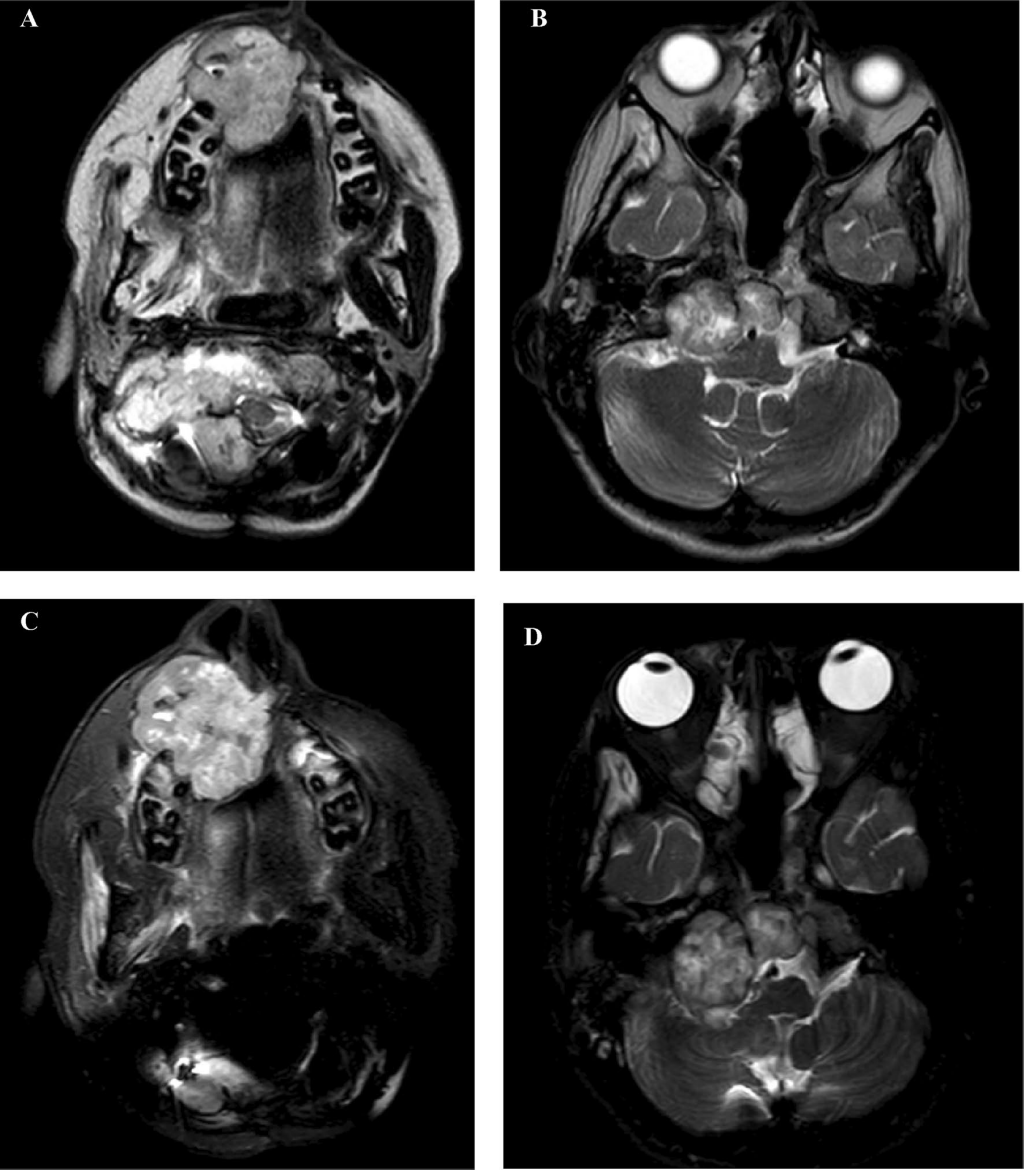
Case 3 MRI Brain. Axial T2 MRI sequences of patient with chordoma; (A,B) before starting pemetrexed 900 mg/m^2^. (C,D) after 3 cycles of pemetrexed with slow growth.
